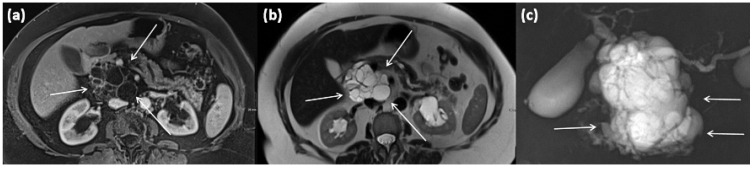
MRI of a 79-year-old female patient with axial T1w (a) after gadolinium-based contrast administration, T2w, and (b) magnetic resonance cholangiopancreaticography (MRCP) (c). A merging and communicating multicystic formation is seen at the head of the pancreas, with the single cystic parts showing marked differences in size. Septa show mild contrast enhancement. In T2w a homogenous hyperintense signal is present. The tumor is marked with arrows in all sequences.
